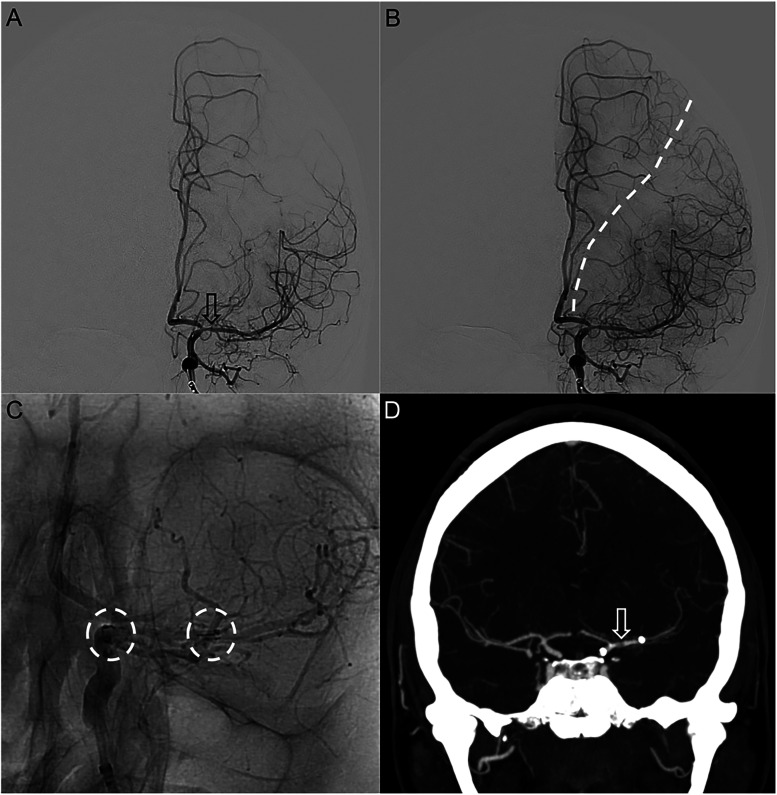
ICA injection of early arterial phase showing significant improvement in caliber of the proximal M1 (arrow) post Neuroform Atlas stenting (a). Late arterial phase demonstrating shift of the watershed towards the 1 o’clock position (dashed line) (b). Additional unsubtracted injection of the left ICA (c) showed the proximal and distal landing zones of the stent, from the distal ICA into the left mid M1 segment. Follow-up CTA after 3 months (d) confirmed remaining patency of the left MCA stent (arrow).
